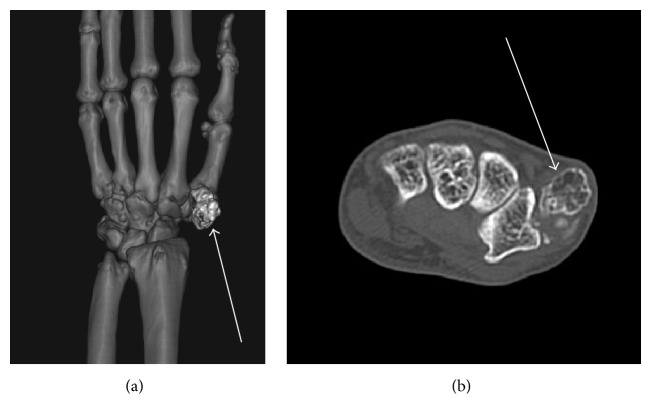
(a) Preoperative CT imaging of the left hand showing a nodule (white arrow) adjacent to trapezium and the base of the first metacarpal bone. (b) Axial section of CT imaging showing the tumor (white arrow).
